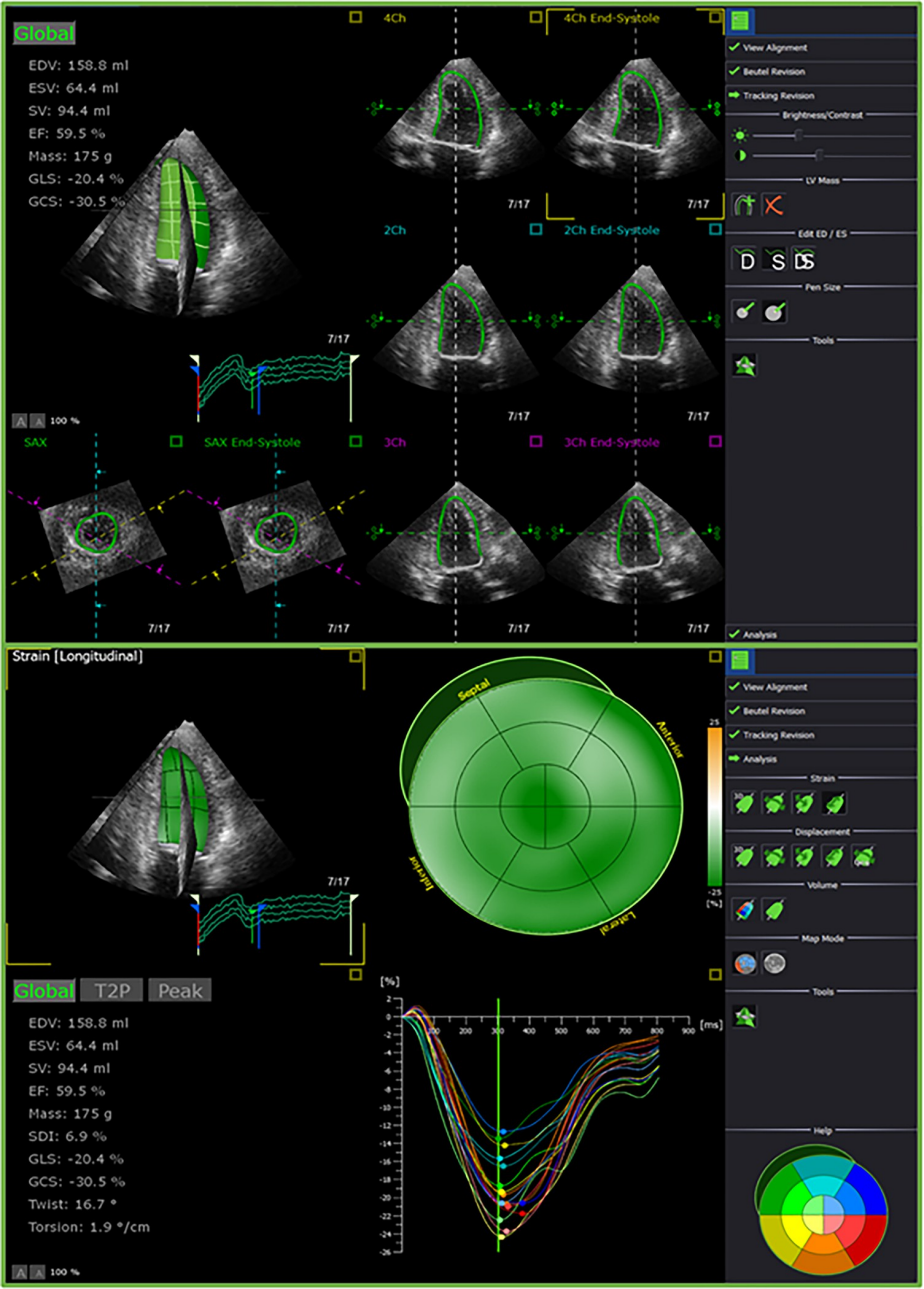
An example of 3D speckle-tracking echocardiography (3D-STE) myocardial deformation analysis performed using TomTec 4D LV-Analysis.
